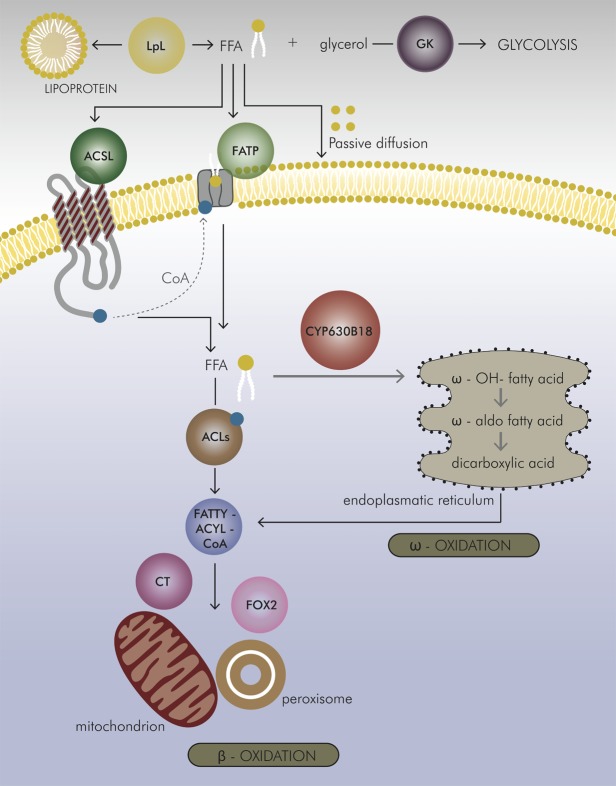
Proposed model for the role of *Gs*CYP630B18 in fatty acid oxidation. The putative involvement of *Gs*CYP630B18 is indicated by grey arrows. Triglycerides from the host plant in the lipoprotein are broken down to free fatty acids (FFA) and glycerol by the action of the lipoprotein lipase (LpL) [57]. Glycerol is further phosphorylated by glycerol kinase (GK) [58, 59] and enters glycolysis. On the cytosolic site, FFAs are activated and coupled to coenzyme A (CoA) by the catalysis of long-chain fatty acyl-CoA synthetases (ACSLs) [60] or by different fatty acid transporter proteins (FATPs) [61]. The transfer through the plasma membrane occurs by a protein-mediated mechanism. In the cell, FFAs can act at different sub-cellular localizations and have functions in energy generation and storage, membrane synthesis, protein modification, and activation of nuclear transcription factors [62]. Oxidation of FFA in fungi occurs mainly through β-oxidation in the mitochondrial matrix or peroxisomes, or through the ω-oxidation pathway in the endoplasmic reticulum. Several acyl-CoA ligases (ACLs) [63], involved in fatty acid metabolism, then attach CoA to the ends of fatty acids to form fatty-acyl-CoA. Fatty-acyl-CoA can pass through the outer mitochondrial membrane, but requires carnitine acetyl transferase (CT) [64] to cross the inner membrane. The multifunctional β-oxidation enzyme FOX2[65, 66] was induced in *Gs* mycelia grown on fatty acids, indicating possible peroxisomal oxidation. In ω-oxidation, the hydroxylase reaction is catalyzed by CYP630 (red bubble) and its redox partner CPR 2. Dicarboxylic acids are then subject to further β-oxidation. Gene IDs and respective changes in transcript abundance in *Gs* grown on fatty acids are given in Table 3 [14, 67].
